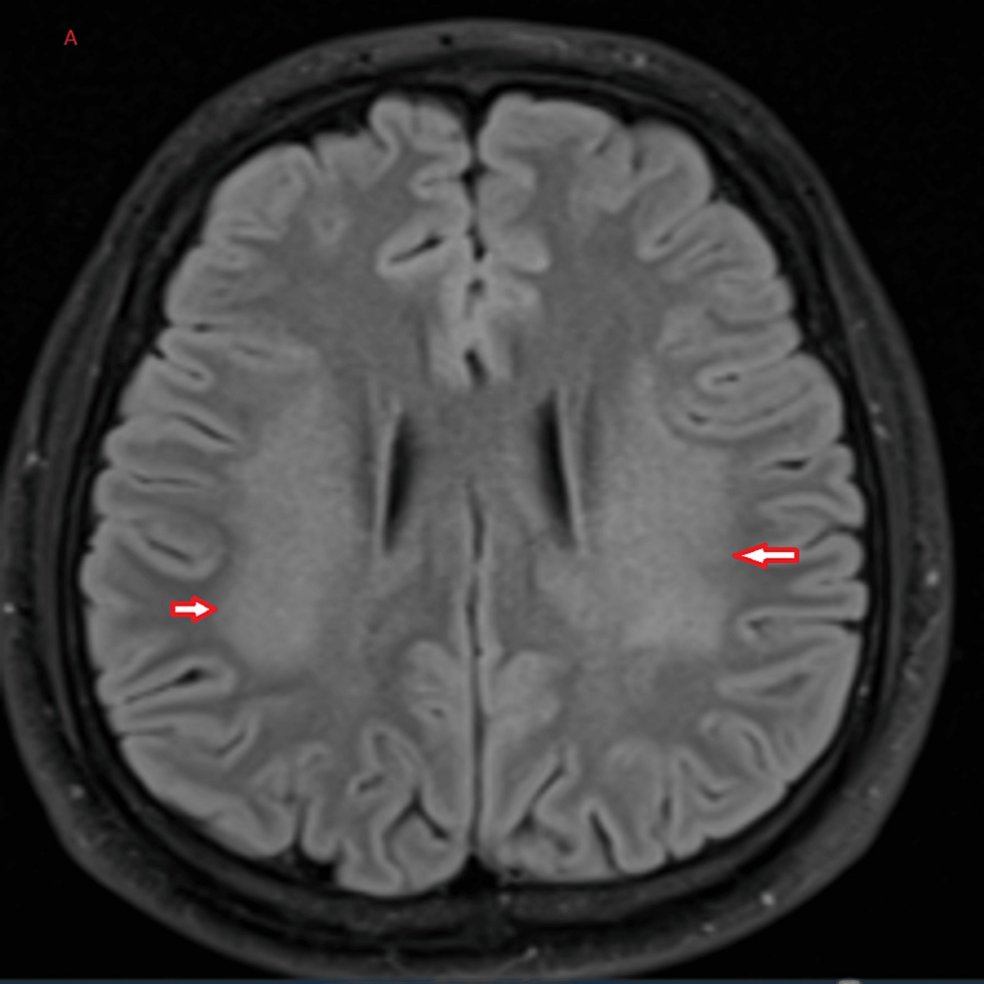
FLAIR hyperintense lesions. The FLAIR MRI sequence, axial cut, shows white matter hyperintense lesions, as indicated by the arrows. FLAIR: Fluid-attenuated inversion recovery.
